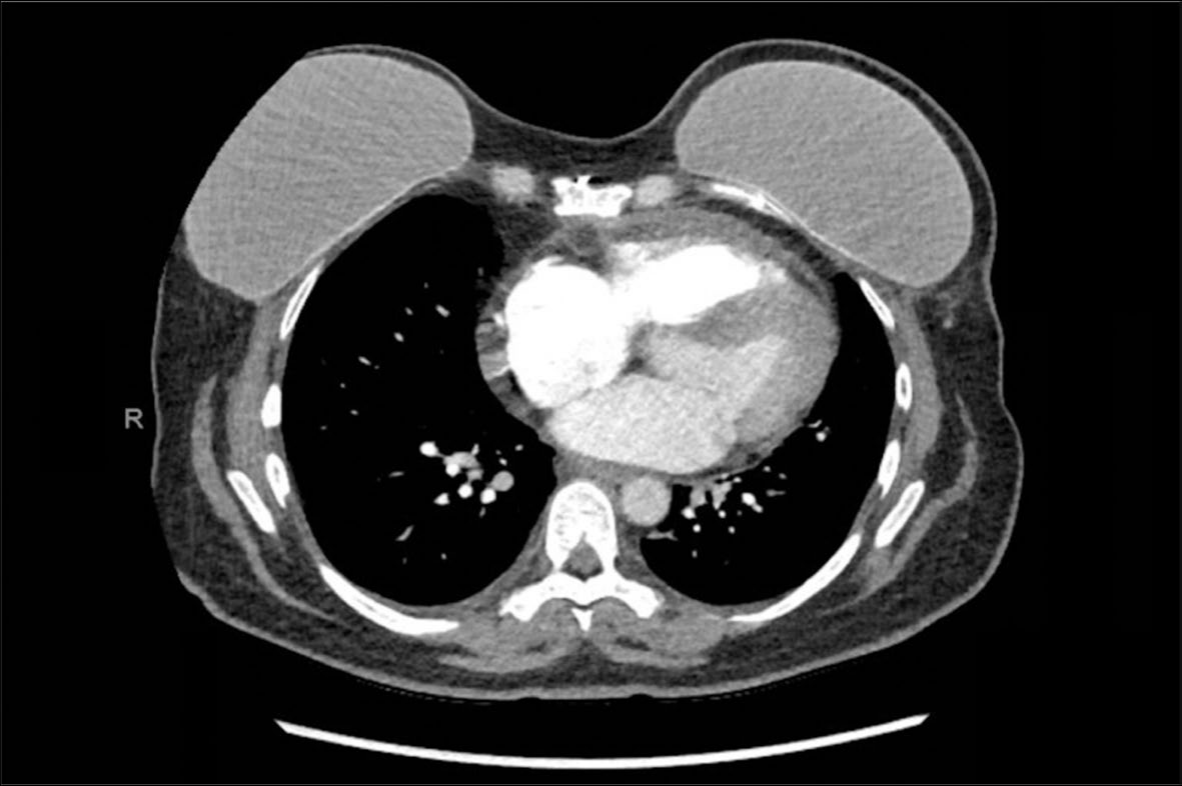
Chest CT showing mild pericardial effusion, no evidence of inflammation or cardiomyopathy
